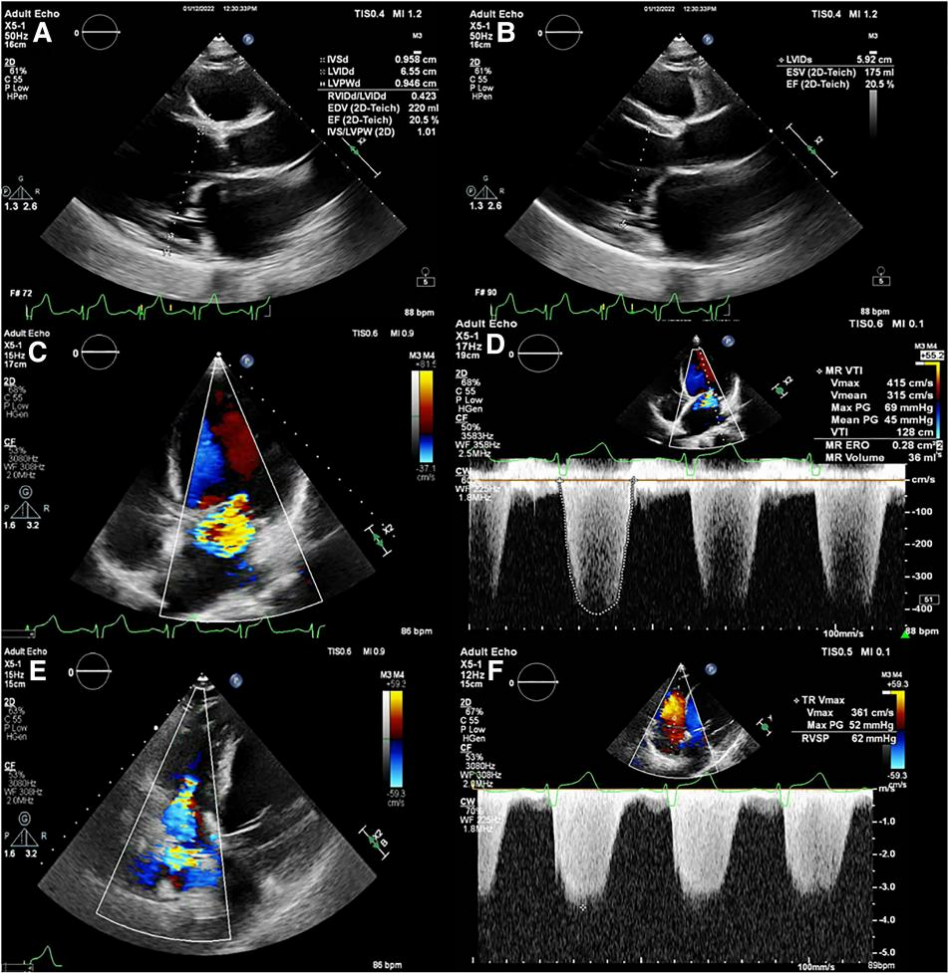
Non-invasive structural and functional echocardiographic assessment prior to IABP placement. LV systolic dysfunction and dilation in diastole (*A*) and systole (*B*). Moderate MR severity assessed by colour (*C*) and continuous flow Doppler (*D*). Moderate TR by colour Doppler (*E*) and peak tricuspid regurgitant velocity estimate of RVSP (*F*).
